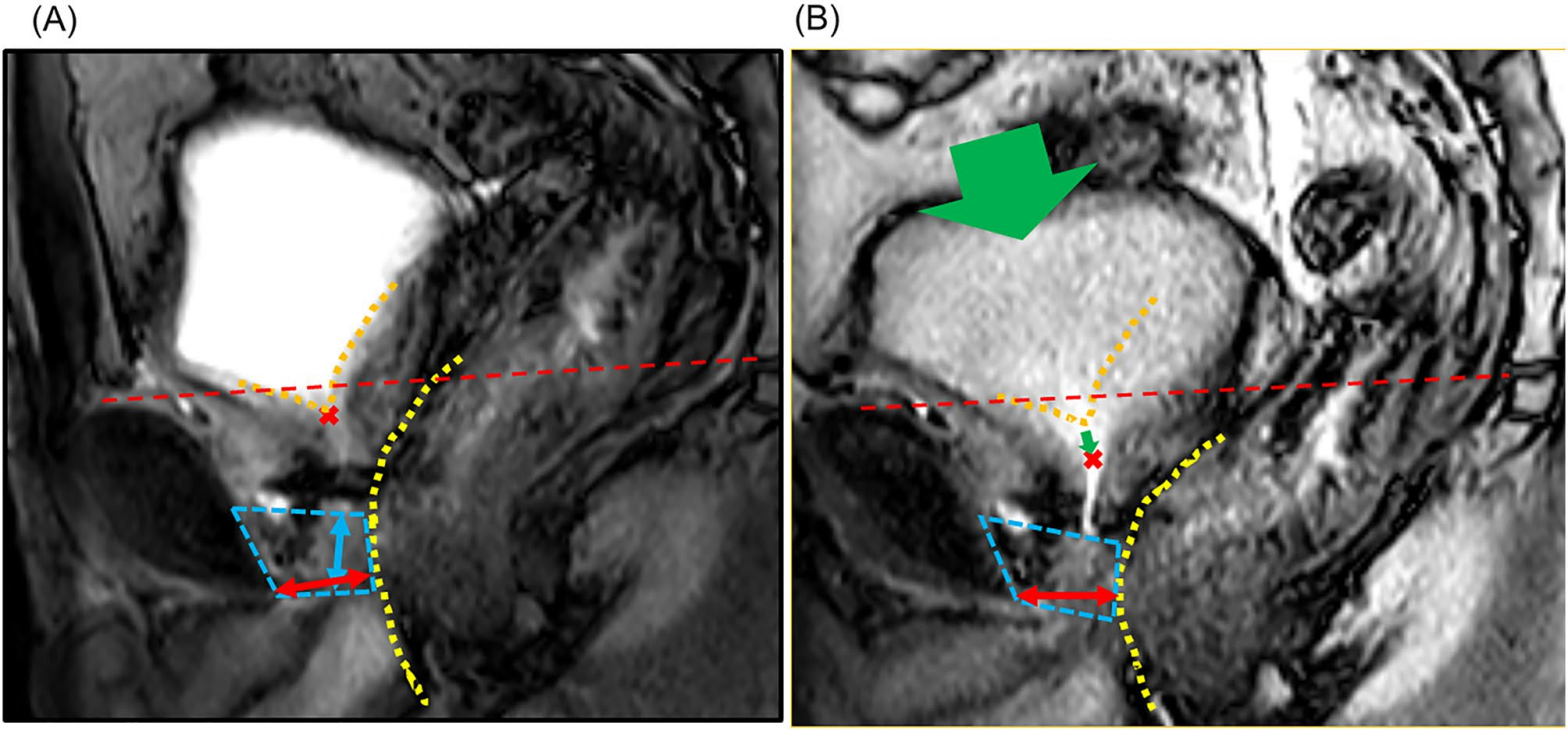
Dynamic mid-sagittal MRI after robot-assisted radical prostatectomy (RARP): at rest (A) and with abdominal pressure (B). The distance from the lowest point of the pubic bone to the anterior edge of the rectal wall (PB-AR, two-headed red arrow) was measured at rest and with abdominal pressure (large green arrow). The external urethral sphincter is indicated by the box surrounded by the blue dashed line. The anterior edge of the rectal wall is indicated by a yellow dashed line. The bladder base at rest is indicated by an orange dashed line. Each red cross is each urethrovesical junction (UVJ) at rest (A) and with abdominal pressure (B). The UVJ is positioned using the line that connects the superior border of the pubic bone and the lower sacrum (red dashed line) as an index. The small green arrow indicates UVJ movement.
